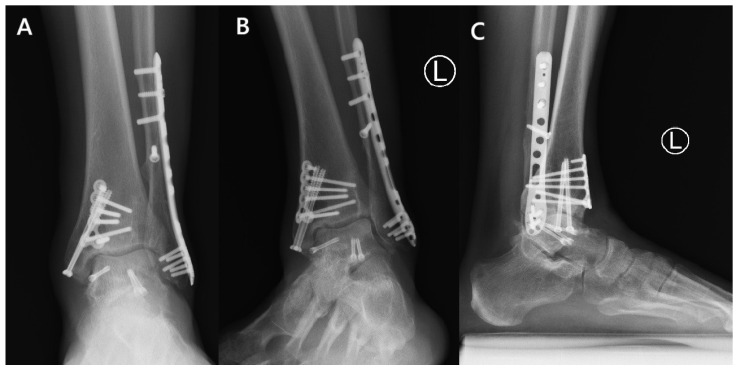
(A) Anteroposterior, (B) mortise, and (C) lateral weight-bearing X-ray views of the ankle at the final follow-up, showing fracture healing without complications but with minor post-traumatic changes.
